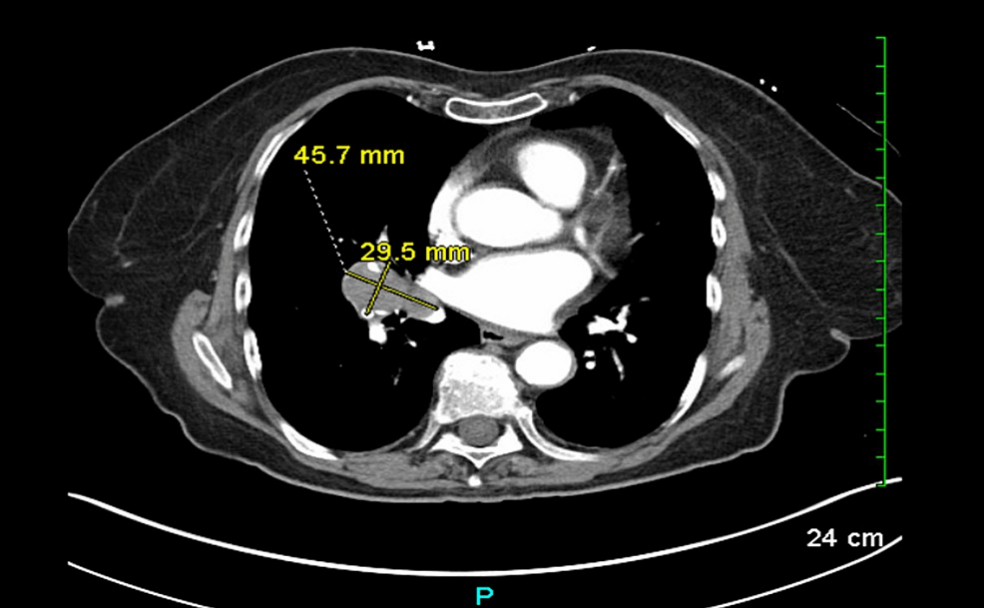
Computed tomography scan of the patient's chest, showing the newly identified lung mass
